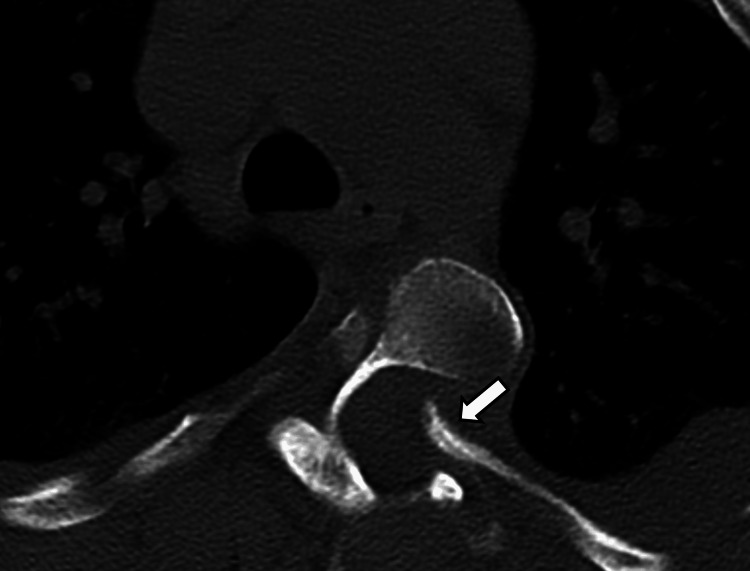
Axial CT image in bone kernel through the T4-T5 neural foramen showing protrusion of the pointed head of the rib through the foramen into the spinal canal (arrow).
